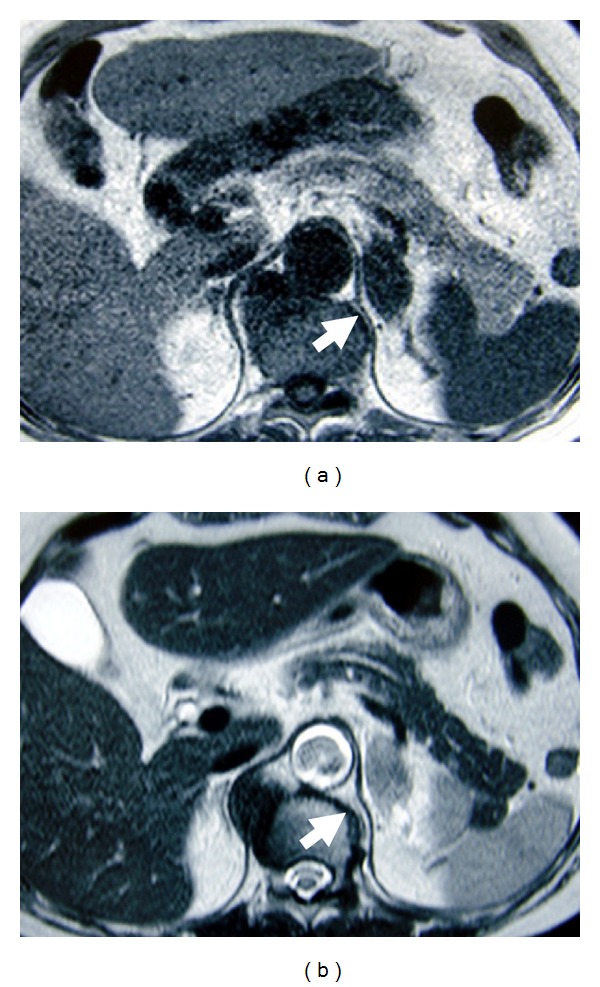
T1-weighted (a) and T2-weighted (b) MRIs of the enlarged left adrenal gland (arrowhead) demonstrating a low- and isointensity signal, respectively. The right adrenal gland was normal in size and shape.
